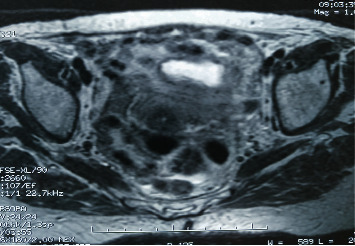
MRI revealing the colouterine fistula.
